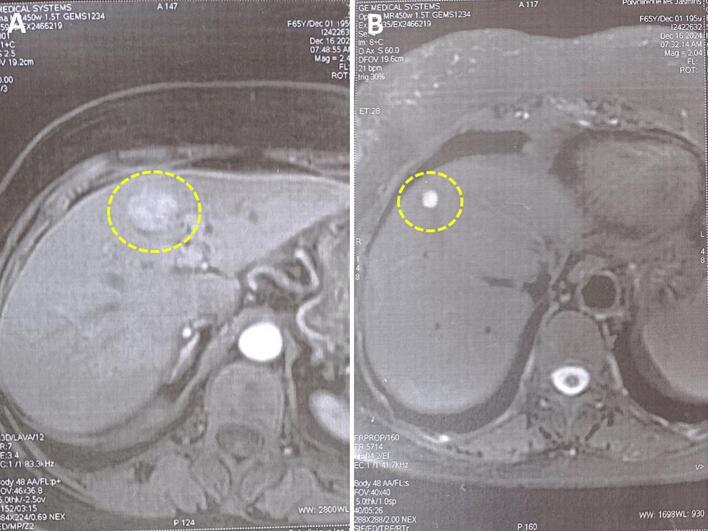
MRI findings of hepatic lesions. (A) Segment IVb shows a roughly rounded nodule with polycyclic margins, demonstrating subtle T2 hyperintensity and isointense T1 signal. (B) Segment IVa contains a non-enhancing 10-mm fluid-intensity lesion, characteristic of a simple biliary cyst.
